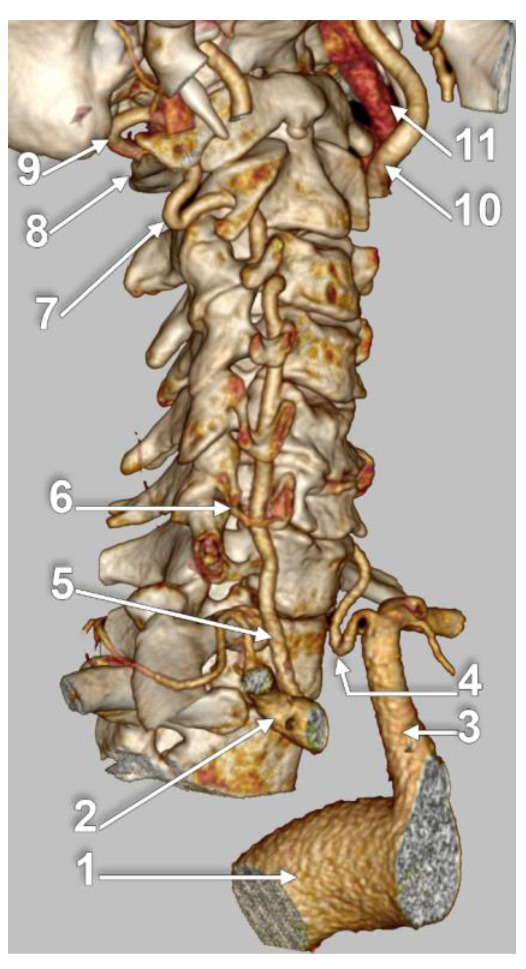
Angio-CT, three-dimensional volume rendering of the vertebral arteries (VAs). Right infero-lateral view. Kinked V1 segment of left VA and V2 segment of right VA: 1. aortic arch; 2. right subclavian artery; 3. left subclavian artery; 4. inferiorly kinked V1 segment of left VA; 5. right VA; 6. right transverse process of C6 vertebra; 7. laterally kinked V2 segment of right VA, in the C1–C2 intertransverse space; 8. posterior arch of atlas; 9. V3 segment of right VA; 10. left internal carotid artery; 11. left internal jugular vein.
